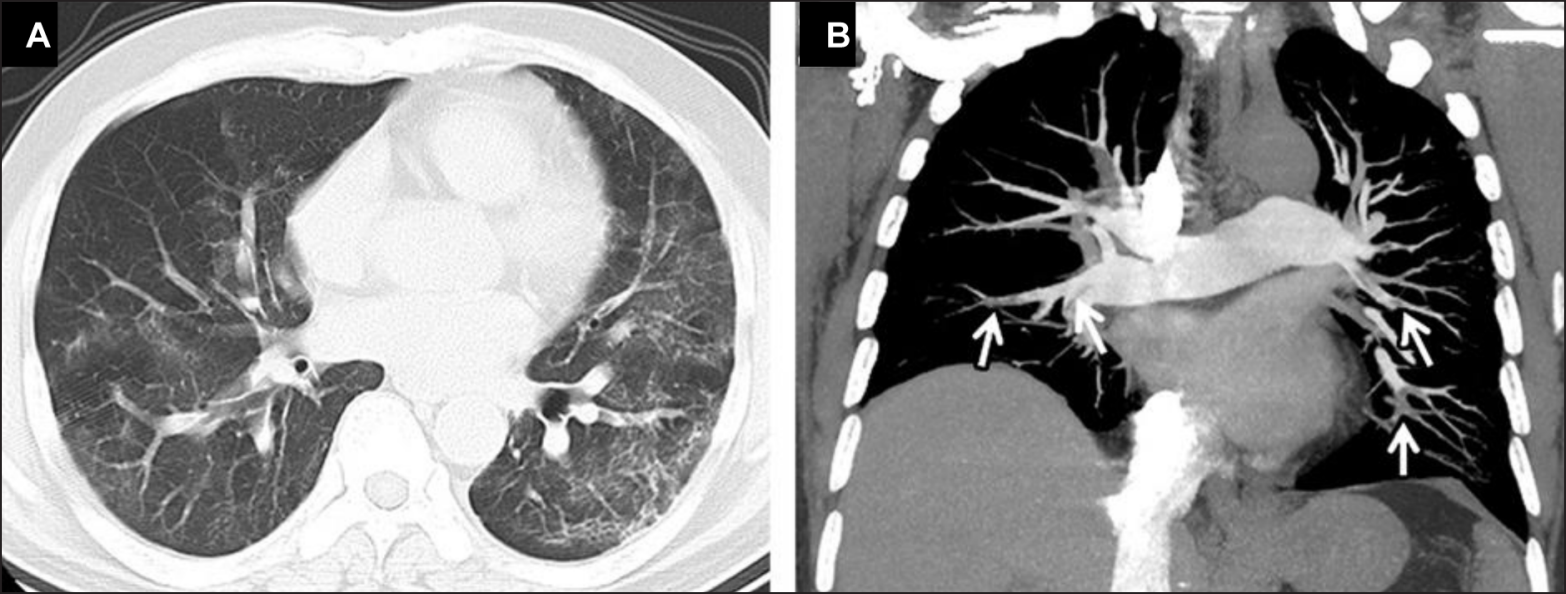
Thoracic CT angiography of a 42-year-old patient with positive PCR for COVID-19. Parenchymal window in the axial section (A) without injection shows peripheral bilateral ‘ground glass’ areas (arrow). The mediastinal window (B) in coronal reconstruction (MIP), reveals several bilateral endoluminal defects involving the lobar, segmental, and sub-segmental branches of the pulmonary artery (arrow).
